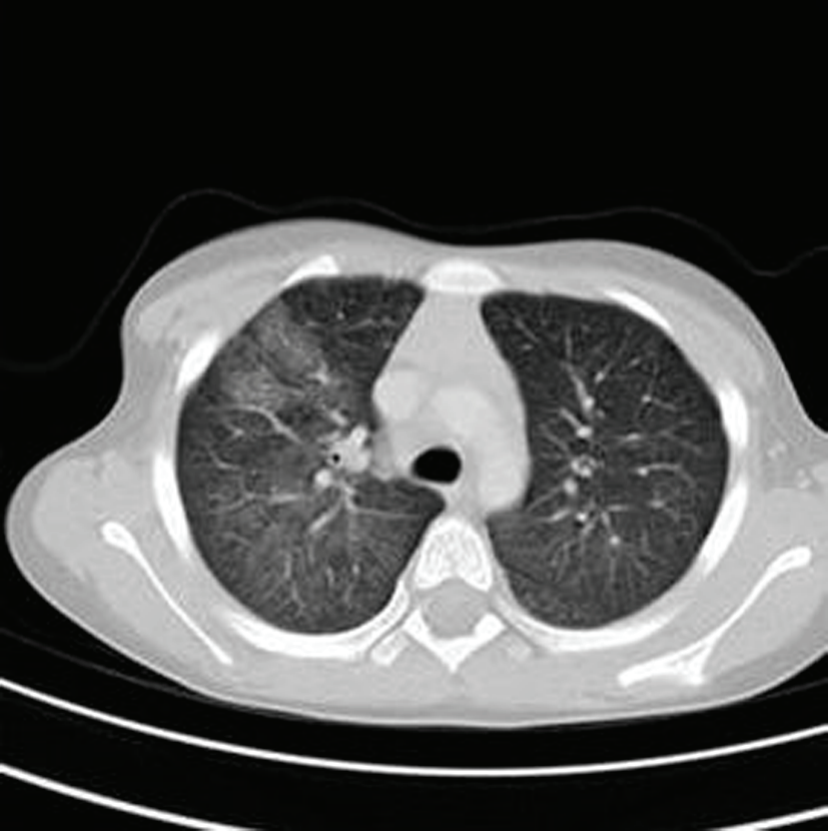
Initial chest CT demonstrated the extent of the airspace filling process. Ground glass opacities were seen in all lobes of the right lung with ground glass opacity identified in the left upper and left lower lobes to a lesser extent. In addition, there is dense right middle lobe consolidation.
